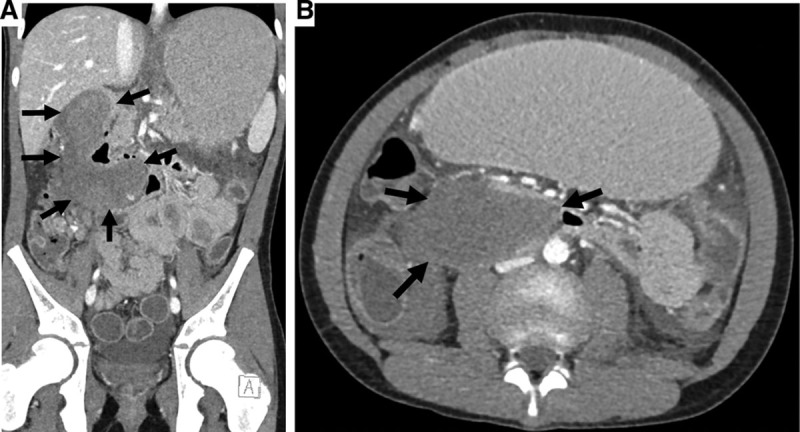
Coronal (A) and transverse (B) computed tomography of the abdomen and pelvis images showing an obstructive dissecting duodenal hematoma involving the second and third portions of the duodenum. Arrows delineating hematoma borders.
